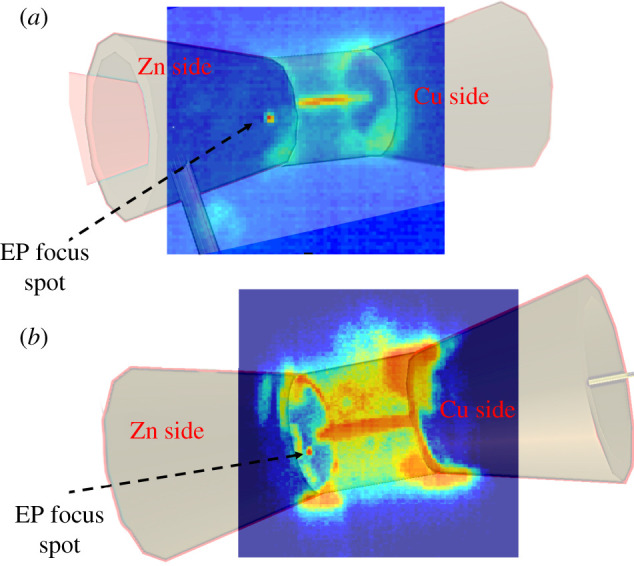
Images from two X-ray pinhole cameras showing two views of the target overlapped with 3D images of the target design. The emission point corresponding to the OMEGA EP laser irradiation is noticeably offset 100 μm away from the centre axis of the cylinder at an angle 30° from the OUESM measurement plane. (*a*) is from port H8C, 117° from the OMEGA EP propagation direction, while (*b*) is from port H13C, at 72°. (Online version in colour.)
